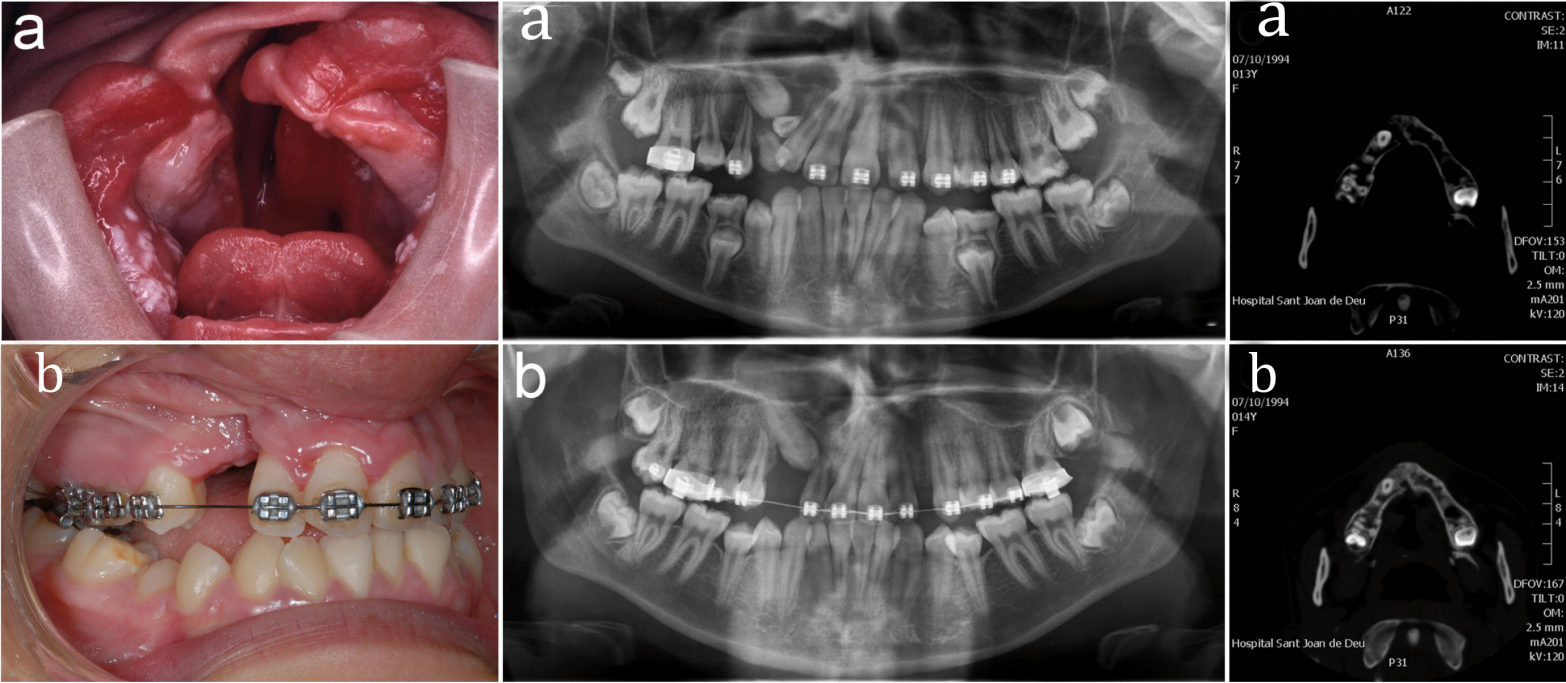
a) clinical and radiographic appearance of the cleft lip and palate b) post-graft clinical and radiographic appearance.
